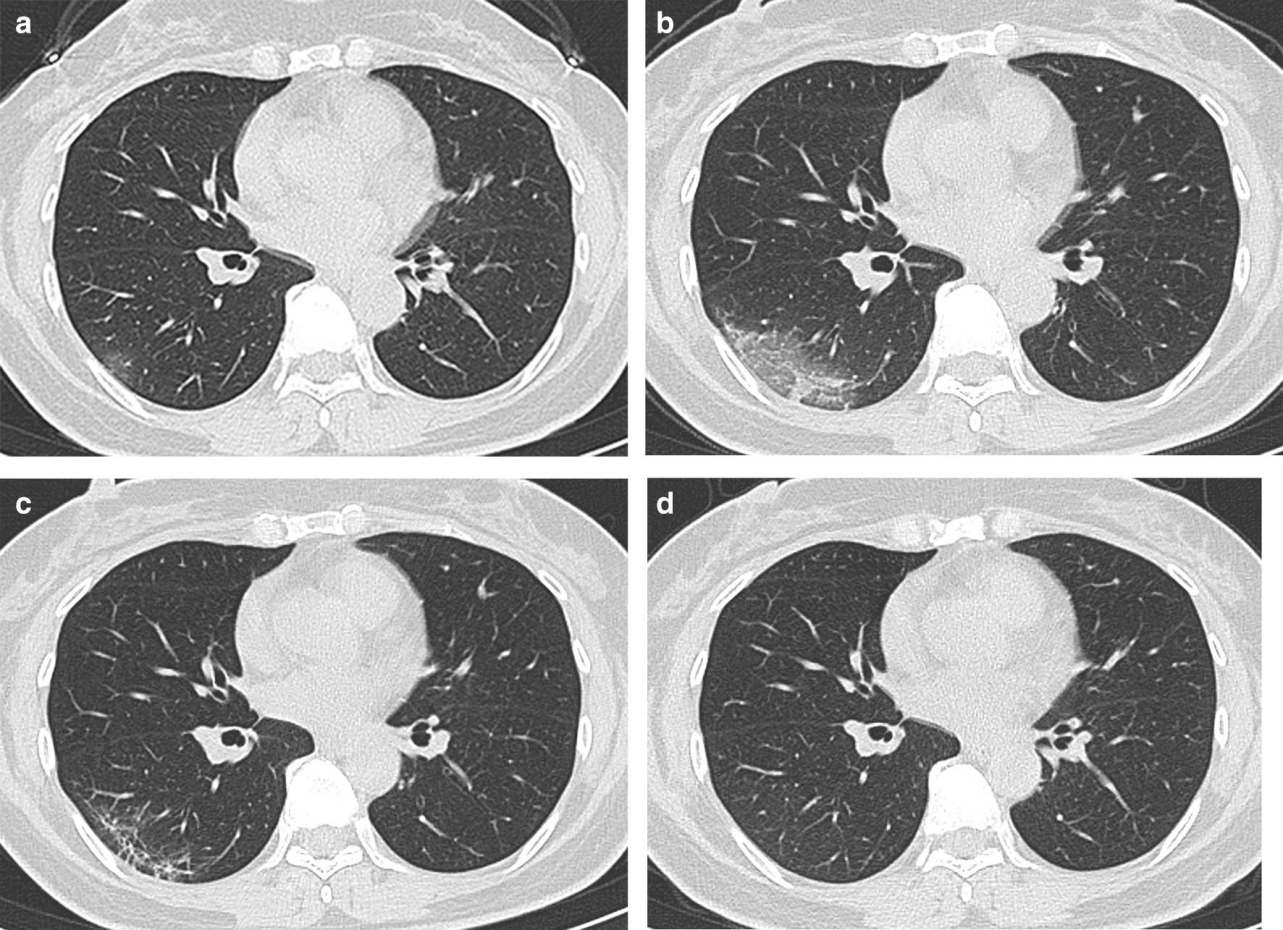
Female, 50-year-old, COVID-19. a The initial CT obtained 4 days from onset of symptoms shows the round ground-glass opacity around the subpleural area of the right lower lobe. b The peak CT obtained 6 days later shows the large patchy ground-glass opacity and a few of consolidation around the subpleural area of the right lower lobe. c The pre-discharge CT obtained 14 days later shows reticulation, irregular lines, and pleura thickening. d. Axial CT. The follow-up CT obtained 64 days later shows a normal chest CT
